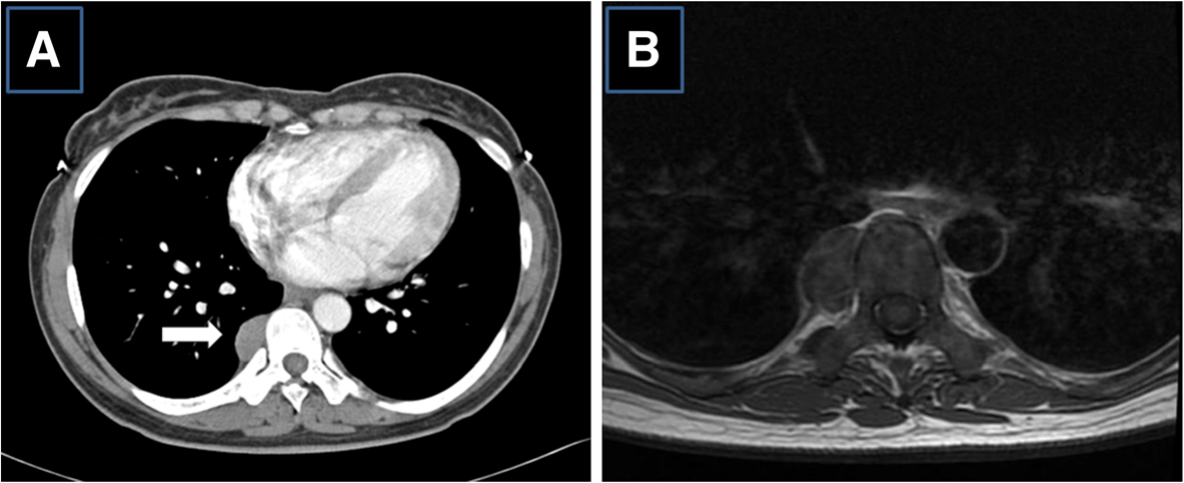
Preoperative images. A) CT scan of the chest demonstrated a paravertebral soft tissue mass (white arrow) through the region Th7 – Th9. B) MRI of the chest could not exclude an invasion into the spinal canal.
